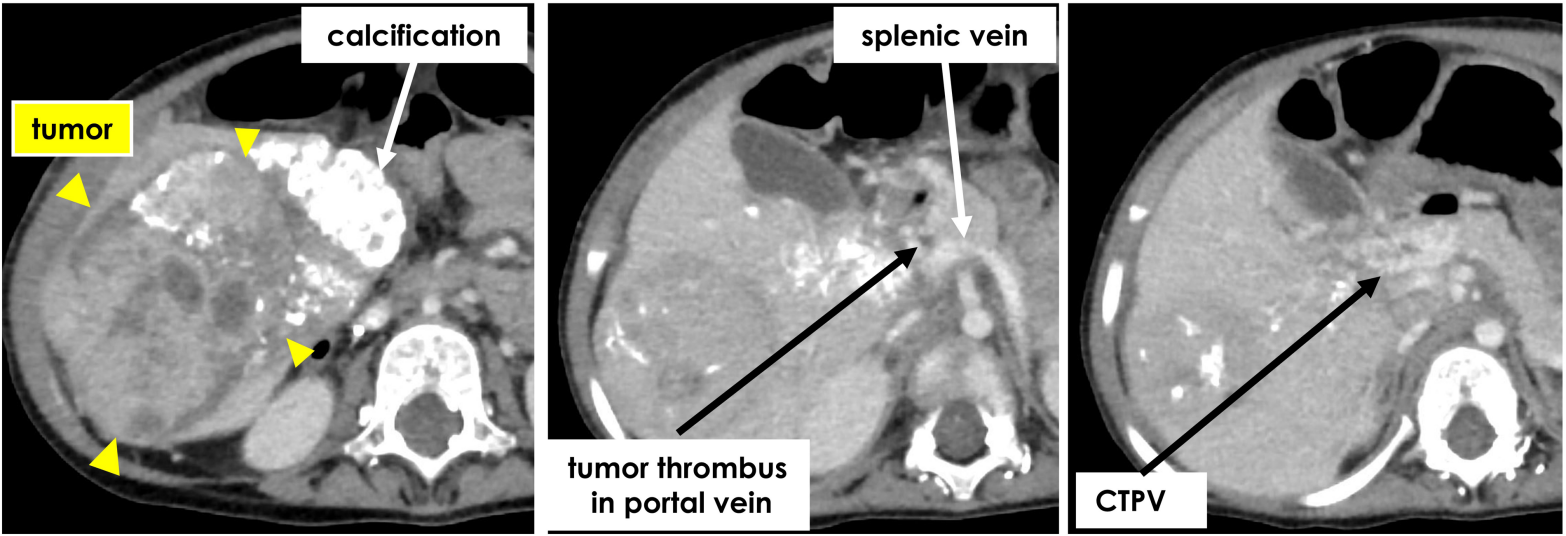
Contrast-enhanced computed tomography after chemotherapy showed tumor thrombus in portal vein trunk and growth of cavernous transformation.
